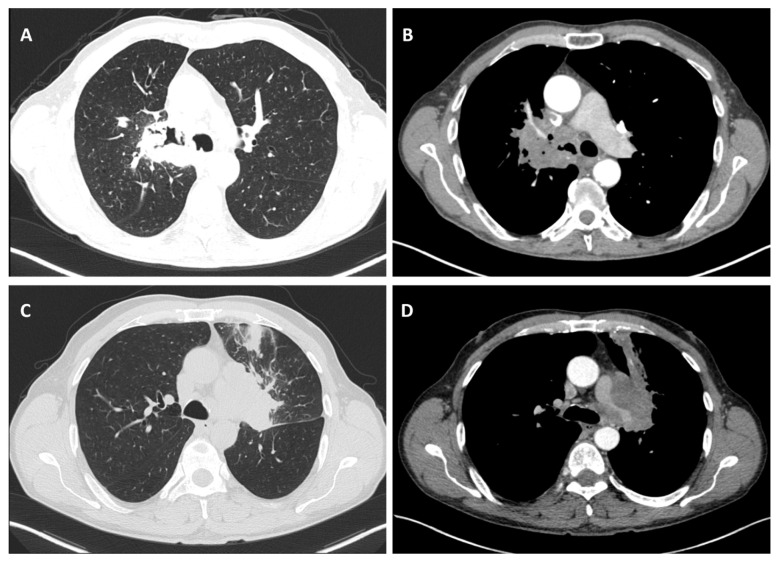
Axial CT of two different cases of squamous cell carcinoma: (A,B) show a right hilar mass with cavity and air bubbles inside, with invasion of the hilar vessels and right main bronchus extending over less than 2 cm from the carina; and (C,D) show a left hilar mass with atelectasis, necrosis, septal thickening, and important invasion of the pulmonary trunk and left pulmonary artery.
